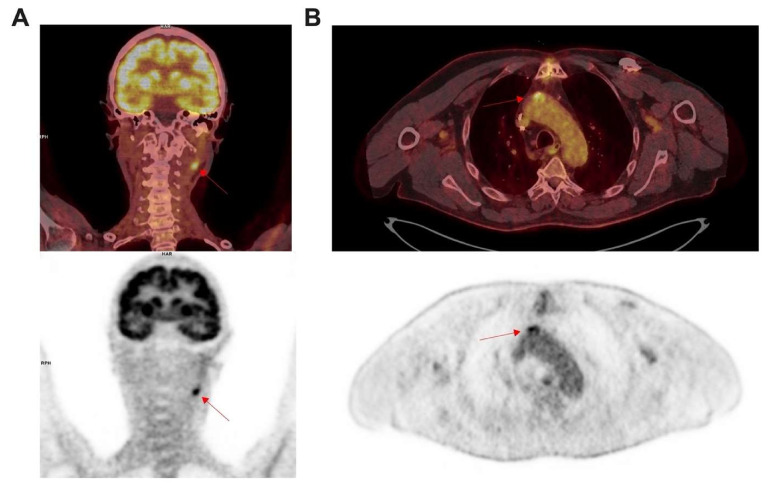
Incidental findings from atherosclerosis in [^18^F]FDG PET/CT imaging. Left panel (A) show coronal images performed for a lung cancer disease in 75 years old women. Intense focal uptake was found ((A) red arrow) corresponding to an inflammatory atherosclerosis process of left internal carotid. Right panel (B) show axial images performed to characterize lung nods in 76 years old patient also followed for a diabetes, tabagism and coronary bypass. Intense focal uptake was found in the ascending aorta ((B) red arrow) corresponding to an inflammatory process in an atherosclerosis plaque with mineralization.
